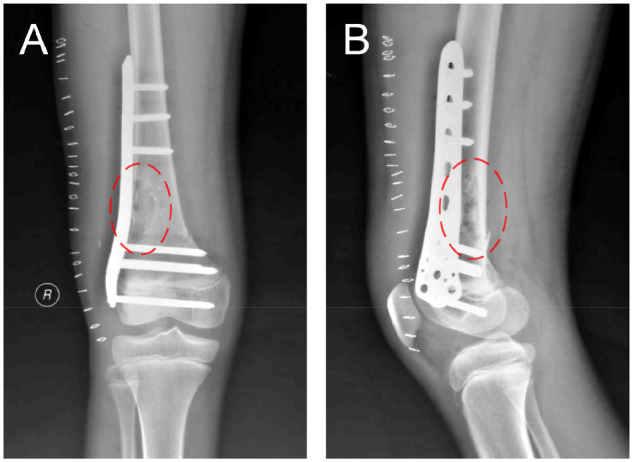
Frontal (A) and lateral (B) views of plain radiography at 3 days after the operation (the surgical site is noted by dashed circle in each picture).
